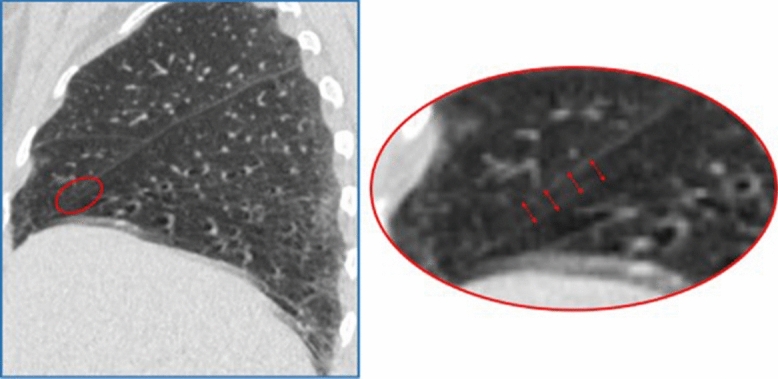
Example of a motion induced blurring artifact in a CT image.
